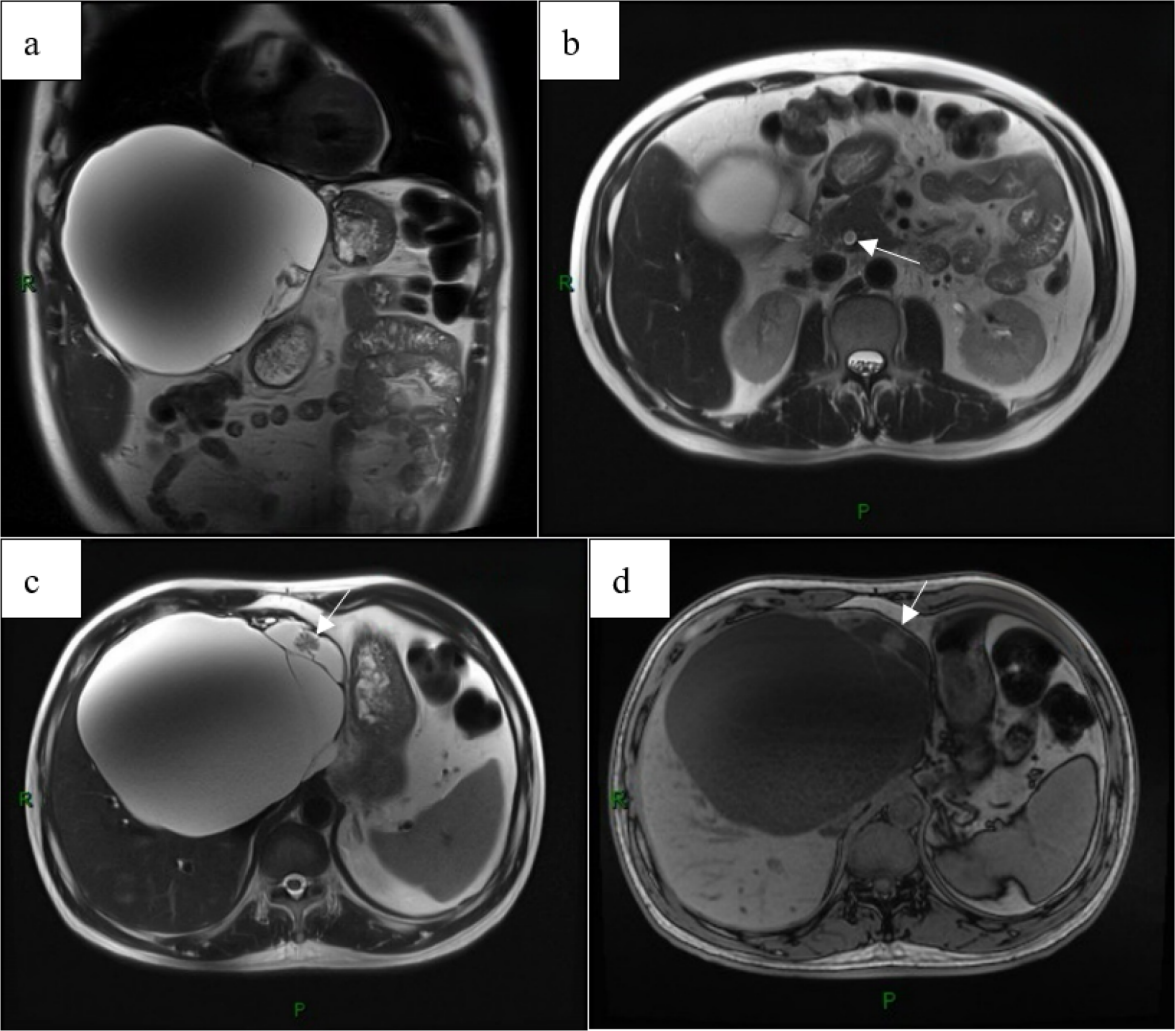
MRI with MRCP demonstrates a large complex cystic mass in the left hepatic lobe measuring approximately 17.3 x 13.9 cm (a), initially suggestive of a giant hepatic cyst. MRCP shows intraluminal filling defects within the common bile duct, consistent with choledocholithiasis (b). Internal septations are present, with a cystic-solid component along the anteromedial aspect of the lesion (c, d), findings suggestive of a mucinous cystic neoplasm.
